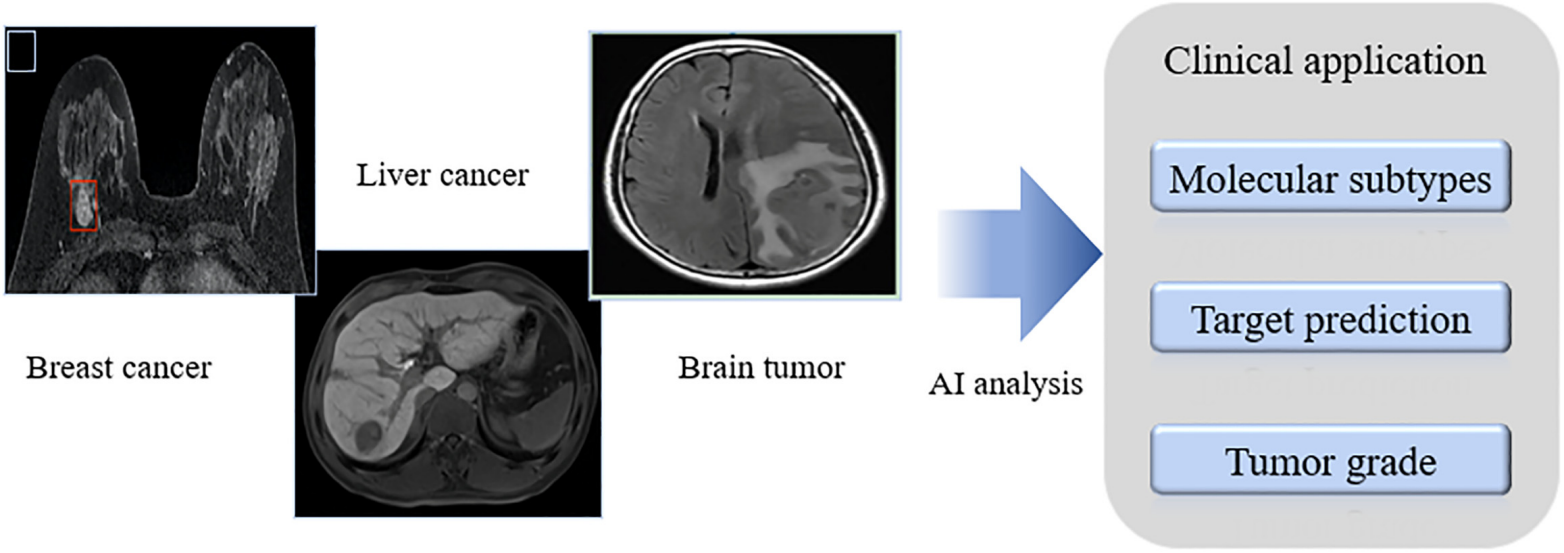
MRI radiomics applied at the molecular level of various tumors.
